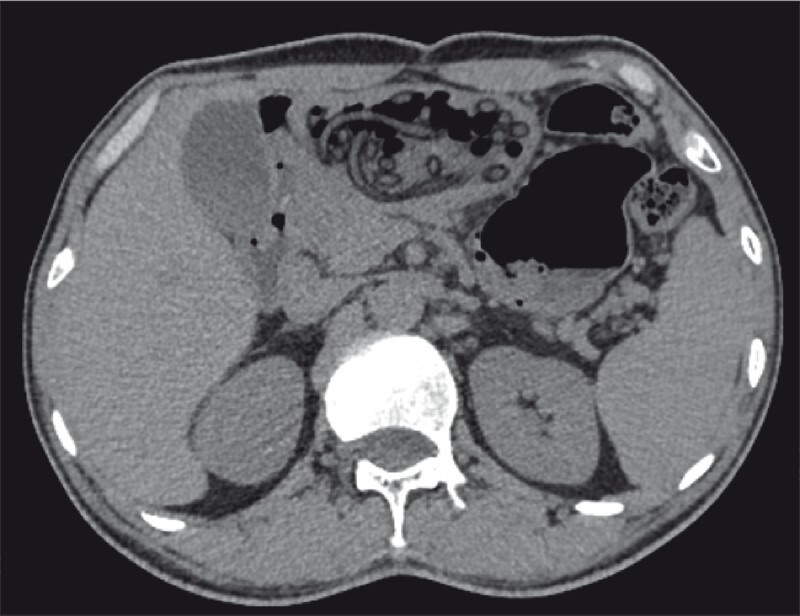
Multiple tubular objects in the stomach and duodenum on the computed tomography scan.
